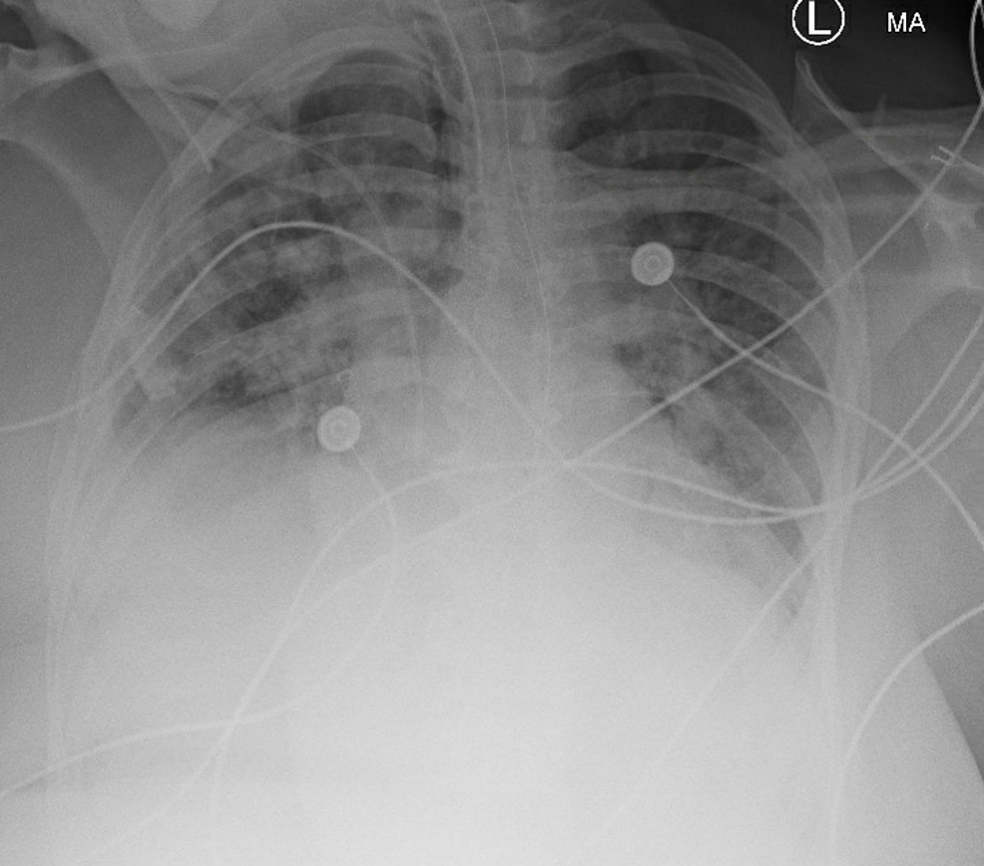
Chest x-ray depicting acute respiratory distress syndrome
